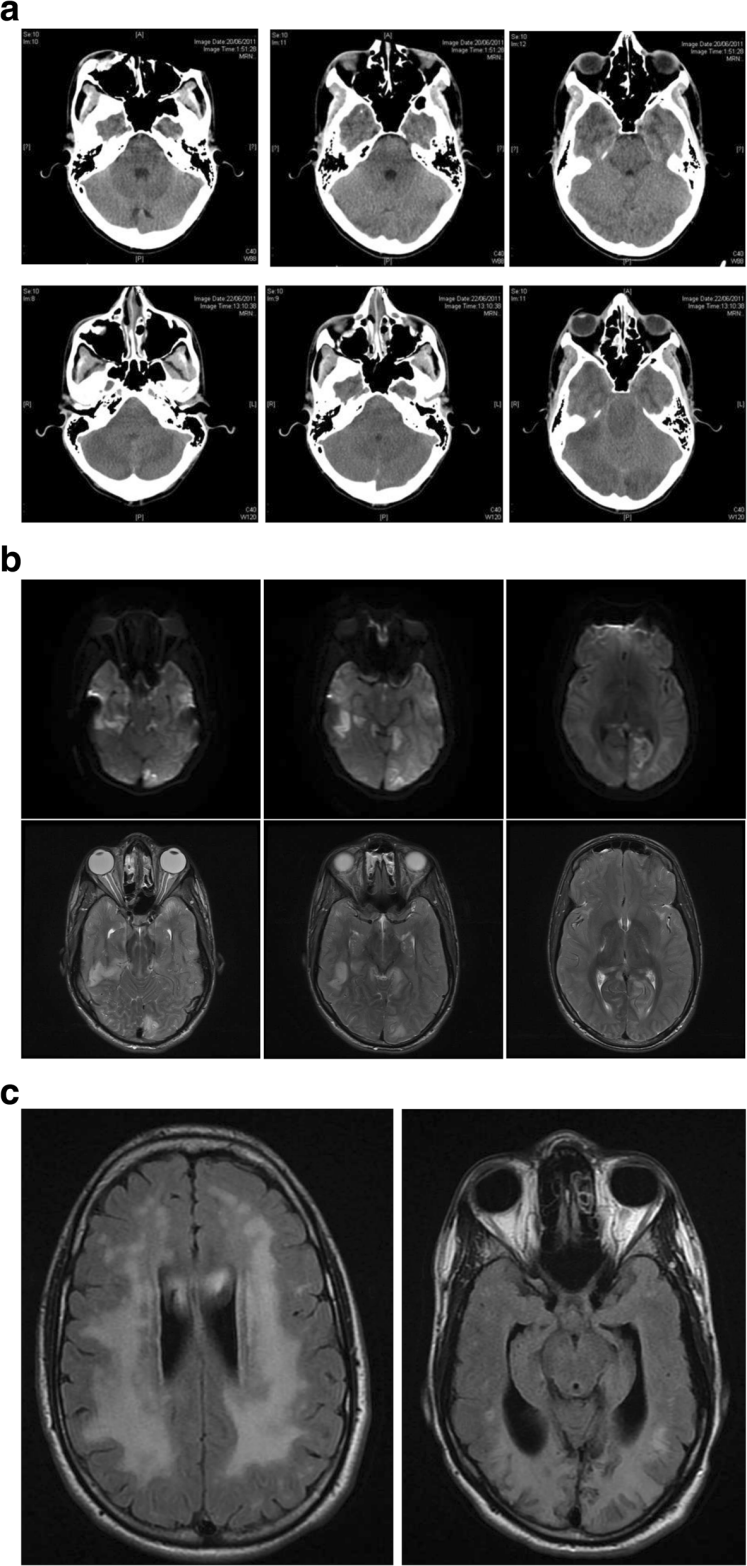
a) CT Scan of 16 yr old boy with HMGCL2D on day 1 of admission (top row) when GCS was 10 and on day 3 (bottom row) when GCS was 3, and pupils were dilated bilaterally indicating deteriorating cerebral oedema. b) Axial DWI (top row) and T2 (bottom row) images of 16 yr old boy with HMGCL2D on day 3 of admission when GCS was 3. There is infarction involving the occipital and temporal lobes, likely secondary to trans-tentorial herniation and compression of the posterior cerebral arteries. Note also the distended optic nerve sheaths and flattening of the posterior globes, in keeping with significantly elevated intracranial pressure. c) Axial T2 Flair images of case 2 with HMGCLD three years after acute life-threatening event (aged 19 years) continuing to demonstrate deep subcortical white matter abnormality with extensive occipital lobe changes leading to cortical blindness
